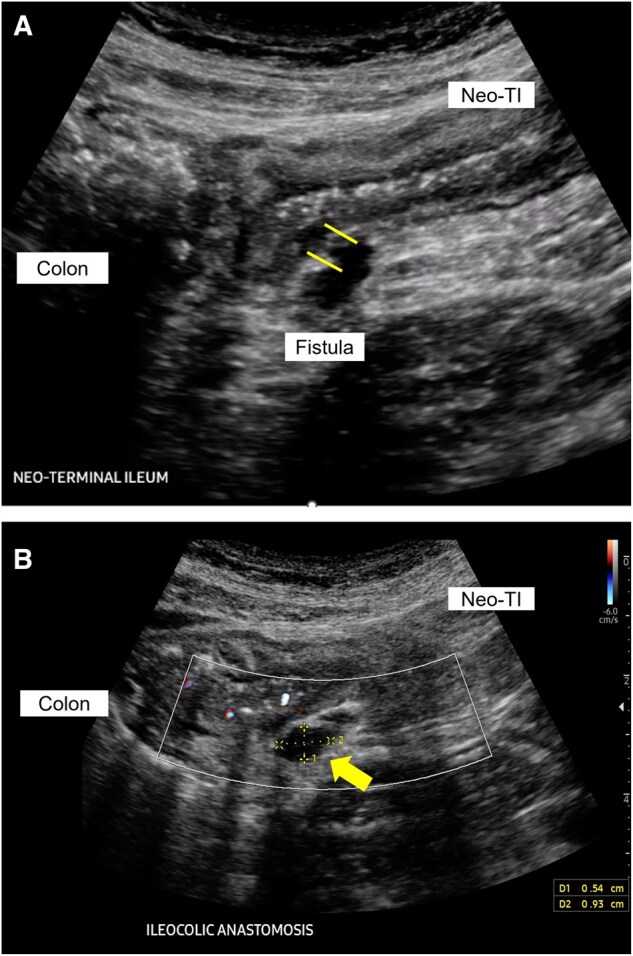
Fistula leading into abscess on IUS. (A) Patient with a fistula (in between lines) arising from the neoterminal ileum (Neo-TI) (B) leading into an intra-abdominal abscess (arrow). There is no color Doppler signal within the dark fluid collection, consistent with an abscess. A cine loop of figure is shown in Supplementary Media 8.
